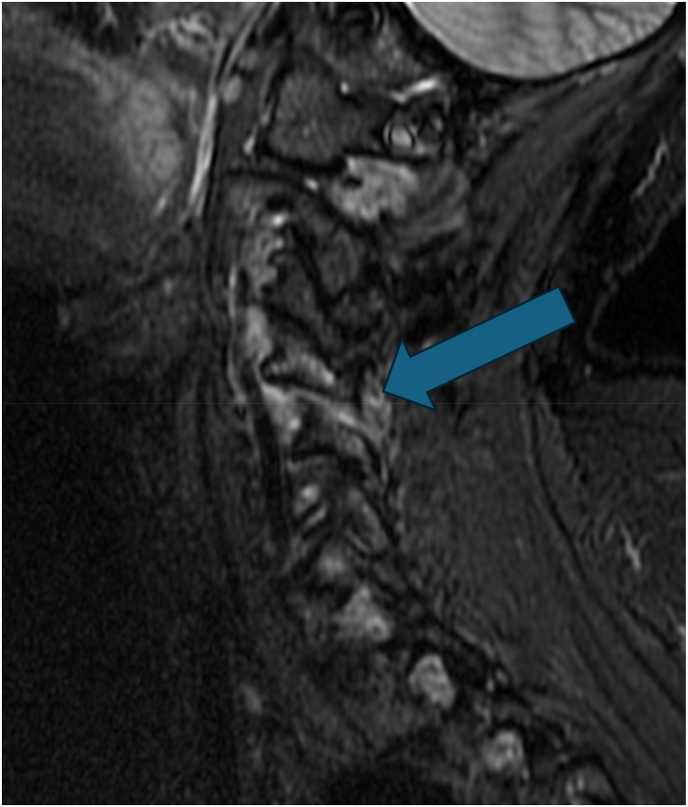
Sagittal STIR MRI with C4-5 peri-facet joint edema (arrow).
